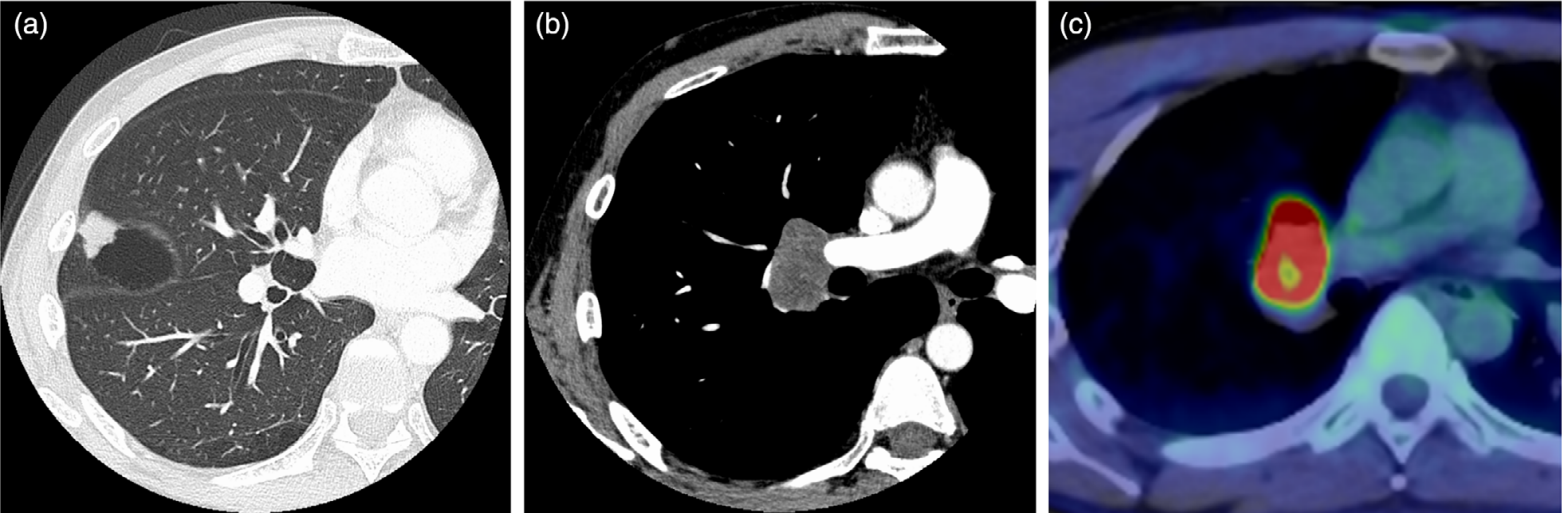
Chest computed tomography (CT) and positron emission tomography (PET)‐CT findings. (a, b) Chest CT revealed a 1.6‐cm‐sized nodule with a pulmonary cyst in the interlobar pleura of right upper and middle lobes and an enlarged #11s lymph node. (c) The lymph node exhibited fluorodeoxyglucose uptake with a maximum standardized uptake value of 17.78 on PET‐CT.
